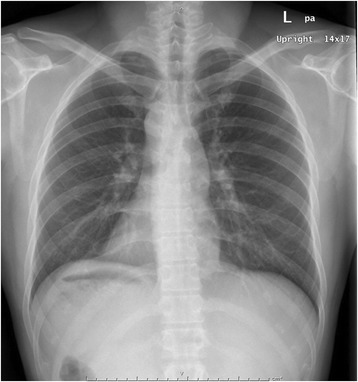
Chest radiograph findings. Dextrocardia is evident
